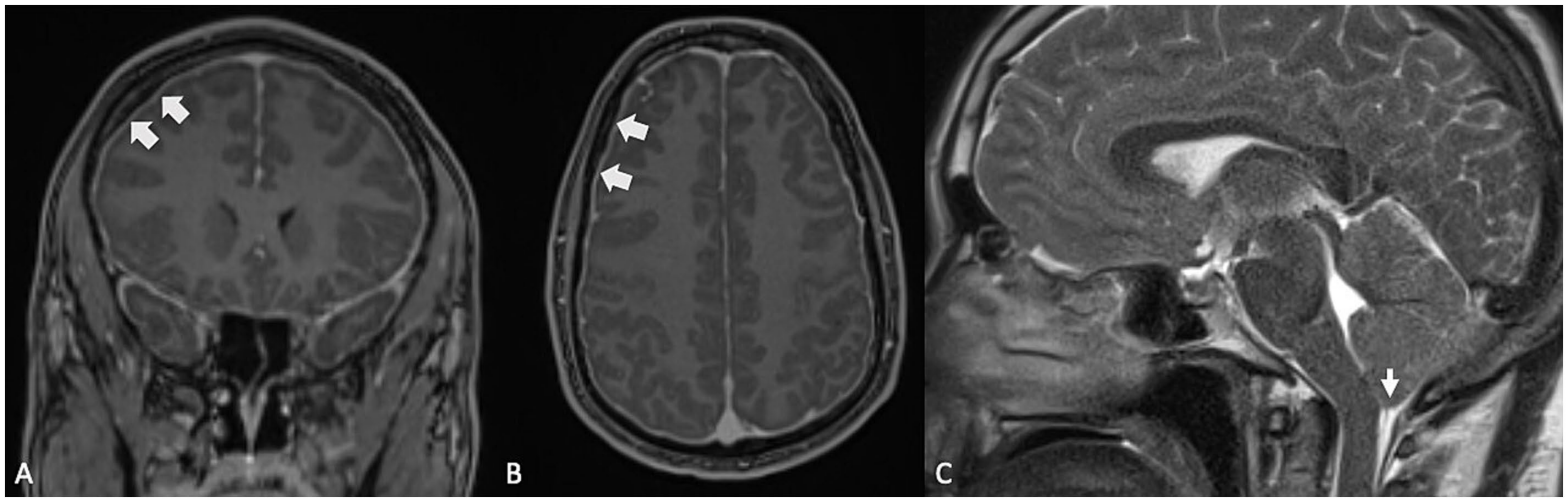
(A,B) Contrast-enhanced MRI, T1-weighted sequences in coronal and axial planes, showing diffuse pachymeningeal enhancement and thickening (large arrows), indicative of intracranial hypotension. (C) Same patient: T2-weighted sequence, sagittal plane, demonstrating moderate sagging brainstem and cerebellar tonsillar descent, also consistent with intracranial hypotension.
